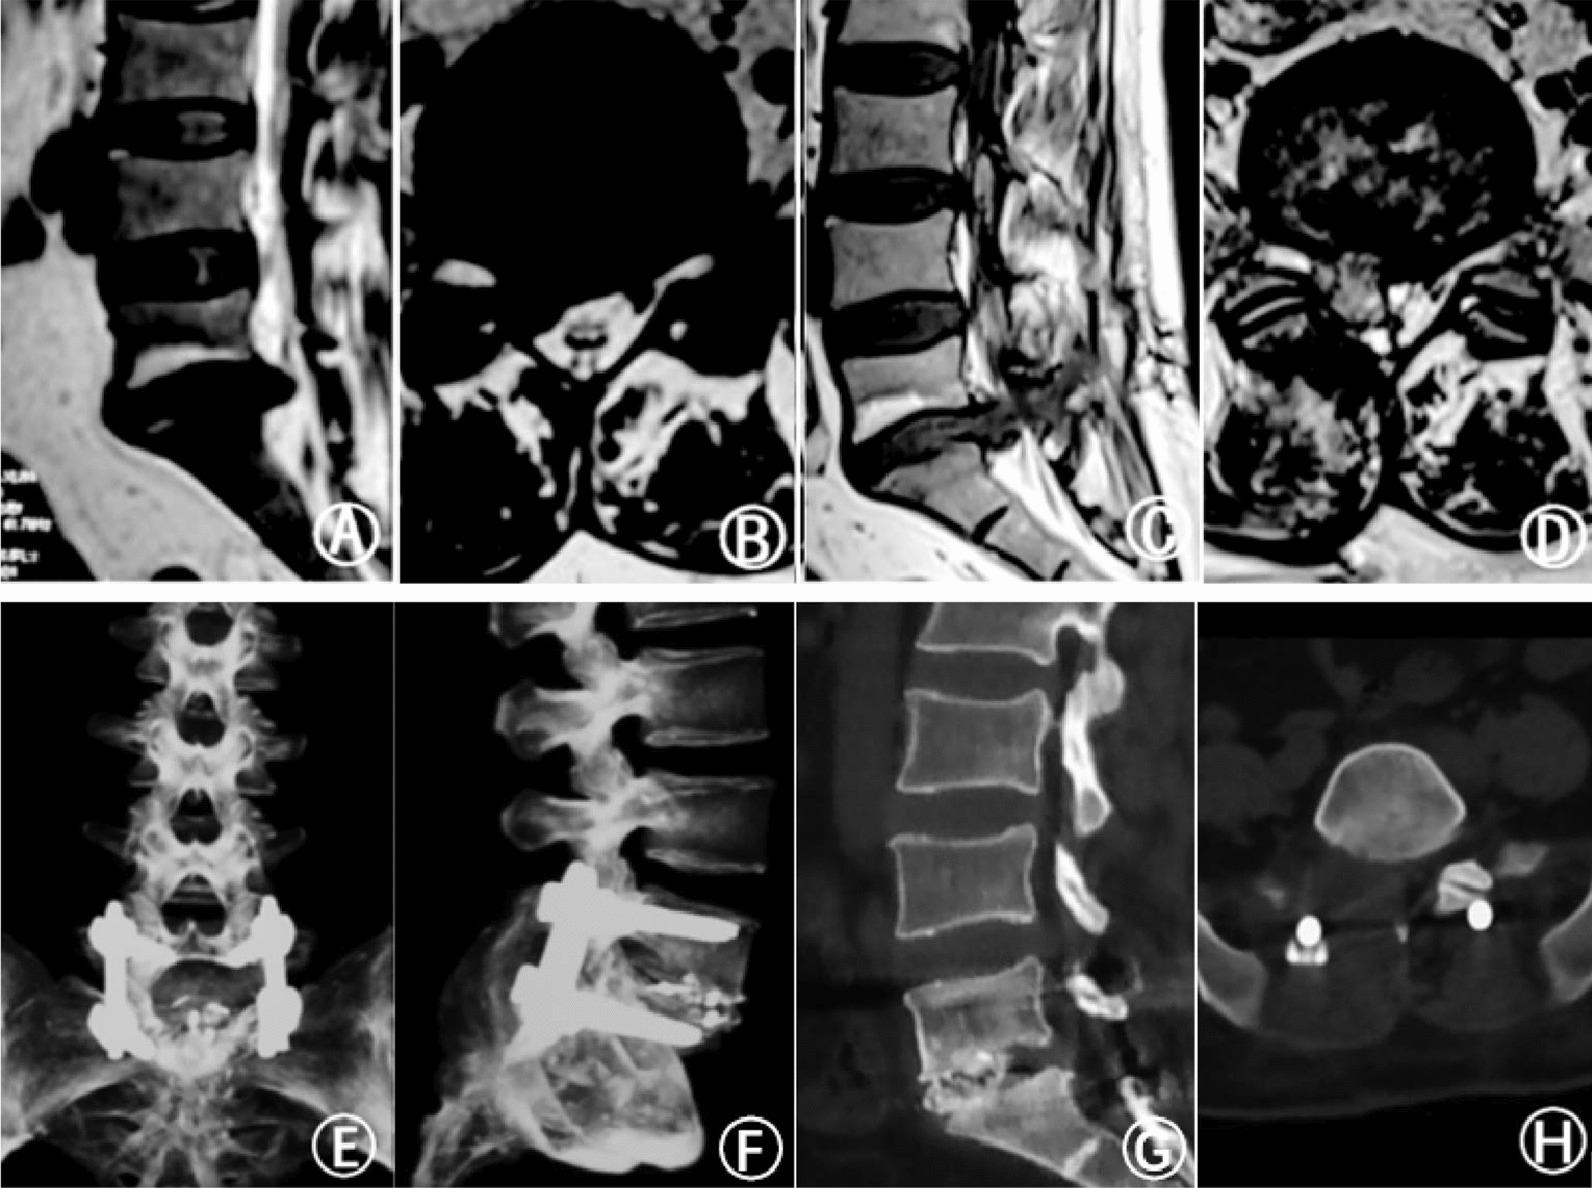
The patient was a 50-year-old female with low back pain and right lower limb pain and numbness for 2 years and was diagnosed with lumbar disc herniation. (AB)First preoperative MRI, patient underwent minimally invasive surgery. (CD)MRI before revision, the patient 's symptoms recurred, and the lumbar lesions and postoperative radiographic changes of lumbar spine can be seen in the figure. (EFGH) Lumbar spine imaging after revision surgery, bone graft fusion was successful
